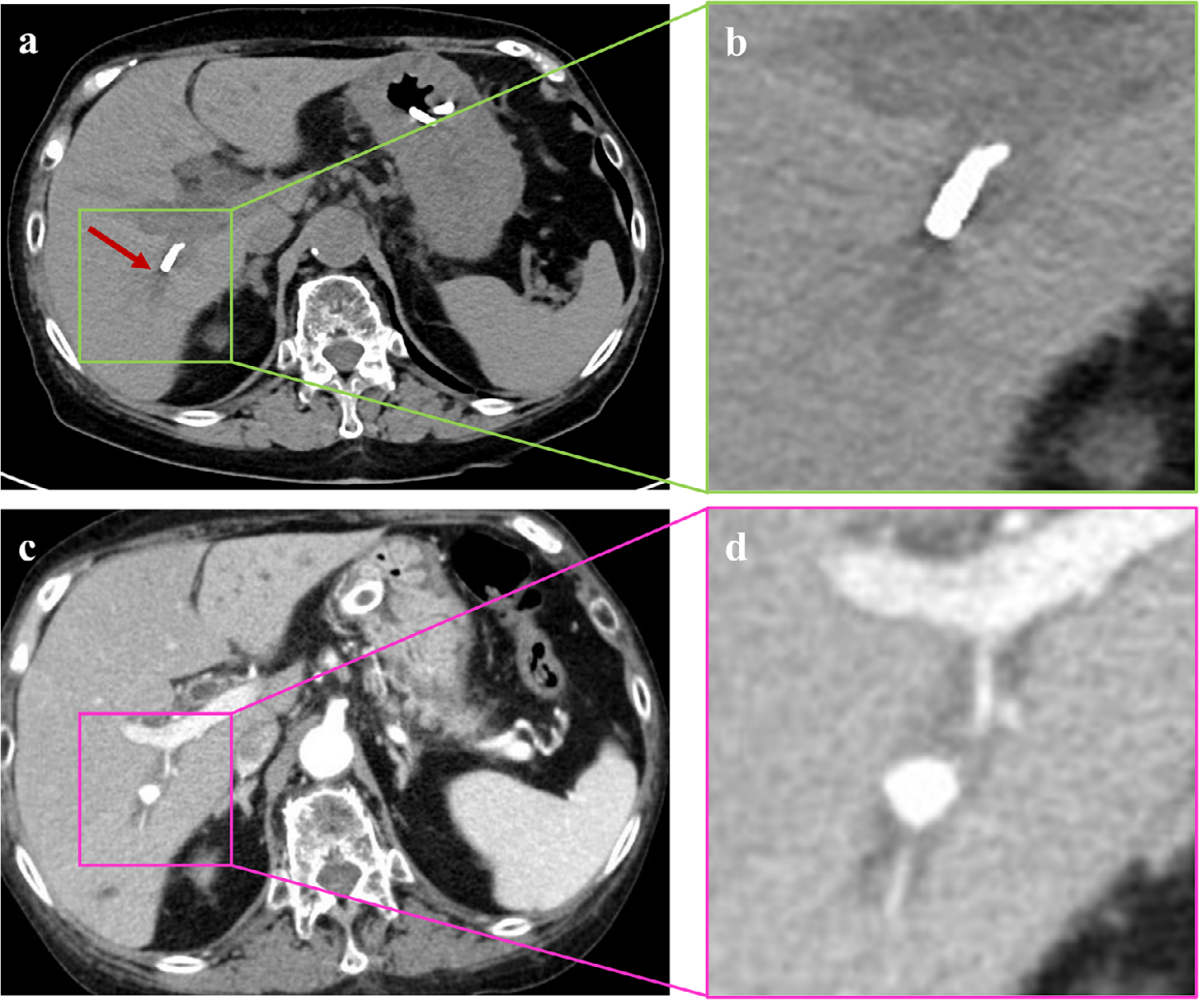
Computed tomography (CT) images on day 46 and day 71 after EUS‐guided hepaticogastrostomy (EUS‐HGS). (a, b) A CT image on 46 days after EUS‐HGS. The hepatic end of the plastic stent had spontaneously migrated and was located in the posterior area of the liver (red arrow). (c), (d) A CT image on 71 days after EUS‐HGS. An 8 mm pseudoaneurysm was observed in the posterior area of the liver, which is a location consistent with the hepatic end of the plastic stent.
